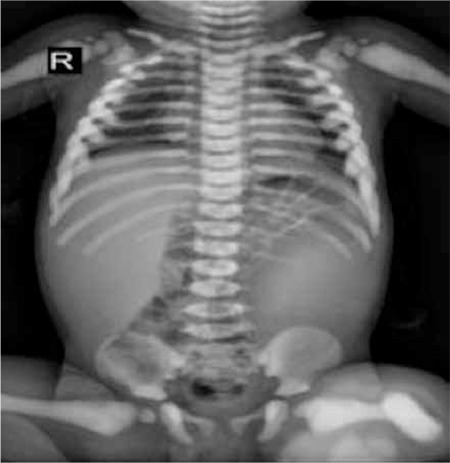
Posteroanterior chest X-ray revealed a uniform increase in bone density.
